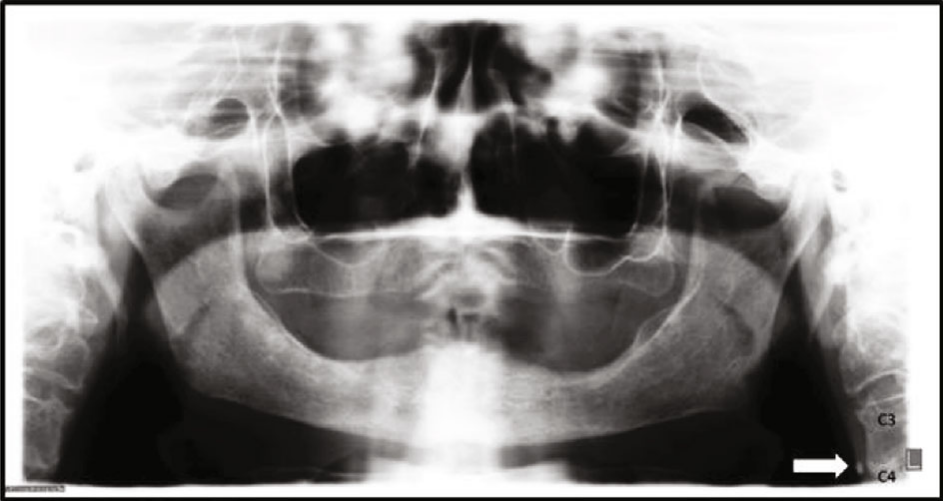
Panoramic radiograph showing radiopaque image circumscribed on the left side of the neck soft tissue region adjacent to the C3 and C4 intervertebral space (arrow).
